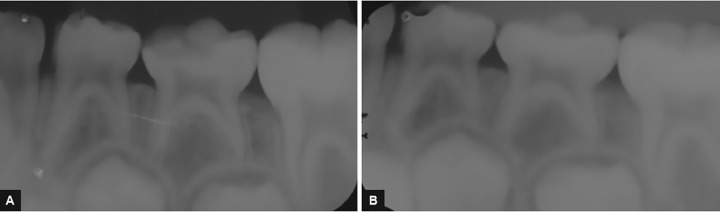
TCR on tooth #75. (A) Initial X-ray; (B) postoperative radiograph in the follow-up period
